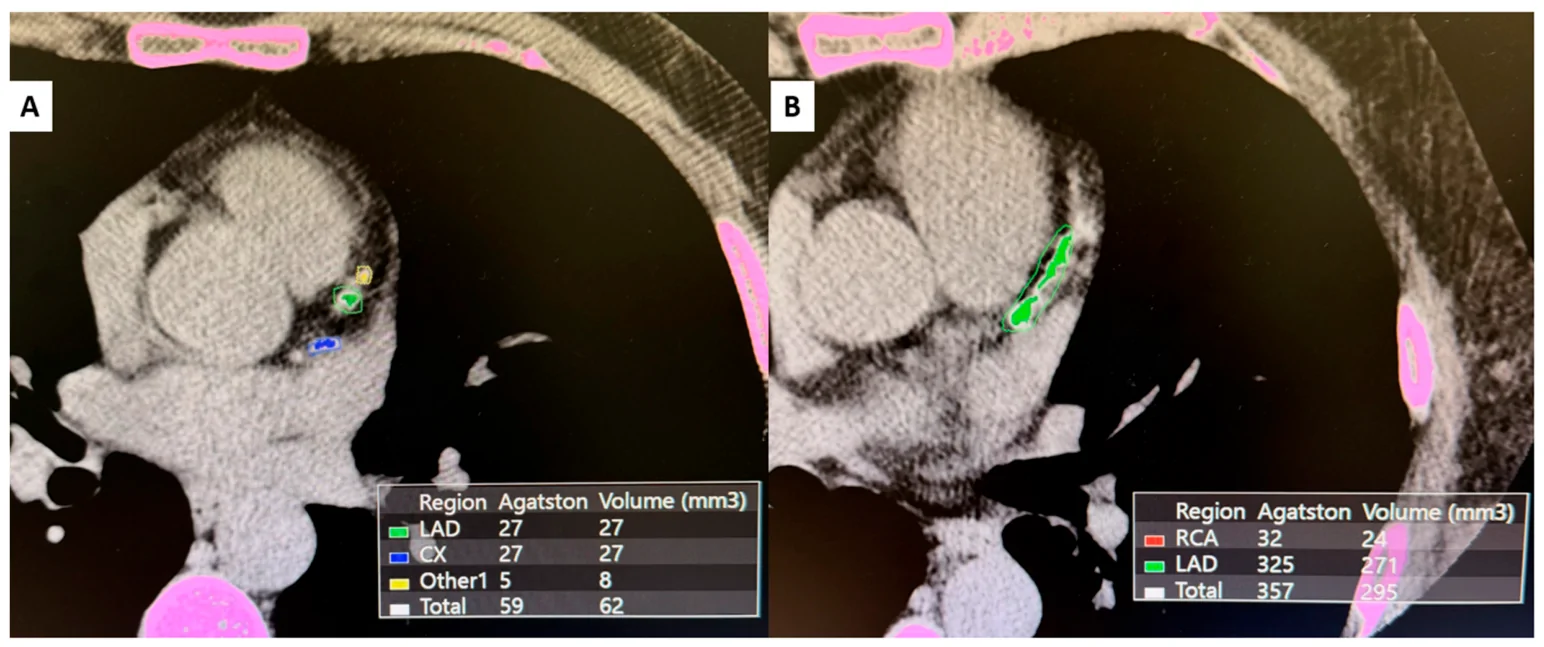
Representative coronary computed tomography images demonstrating coronary artery calcium scoring. (A) Example of mild coronary calcification with low Agatston score. (B) Example of severe coronary calcification with high Agatston score. Color-coded regions indicate calcified plaques in different coronary arteries.
